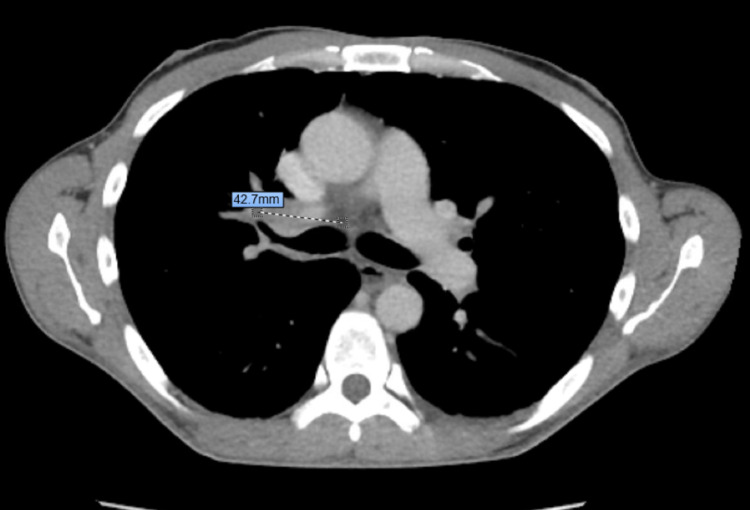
CT of the chest showing a mass (dotted line) compressing the right pulmonary artery and the right main bronchus.
